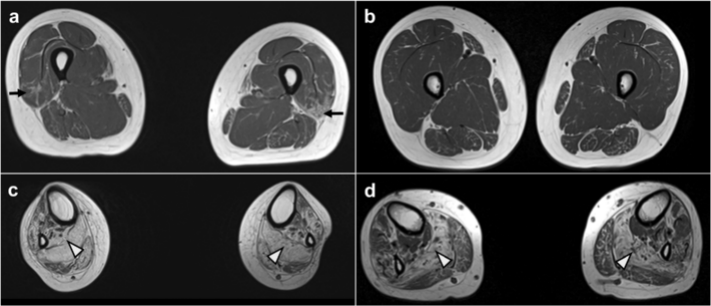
Lower extremity MRI of patients. a, b T1-weighted axial MRIs of the thigh and (c, d) lower leg of patient 1 (a and c) and 2 (b and d). At the thigh level, muscle atrophies and hyperintense signal changes were shown in the vastus lateralis muscle (*arrow*). However, the lower leg MRIs revealed diffuse fatty hyperintense signal changes in the soleus muscles (*arrowhead*), but the tibialis posterior and gastrocnemius muscles were relatively spared
